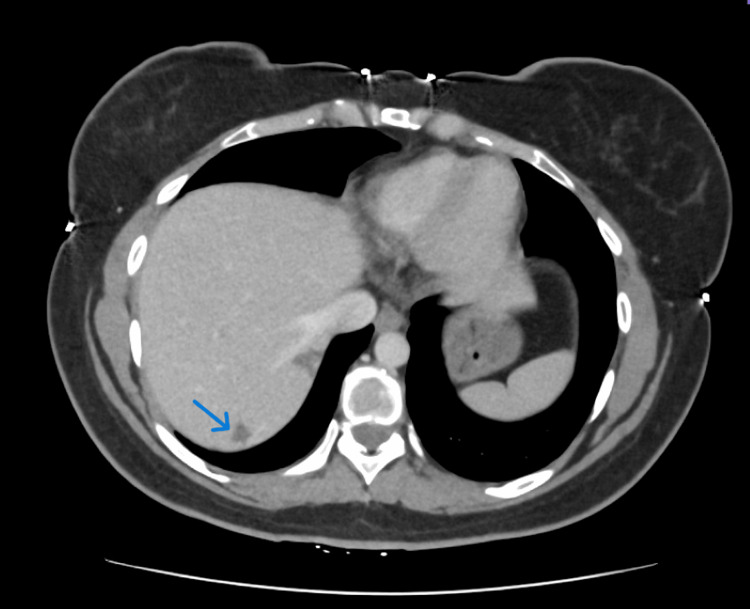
CT abdomen with contrast performed in December 2024 demonstrating cystic lesion in liver (blue arrow)
